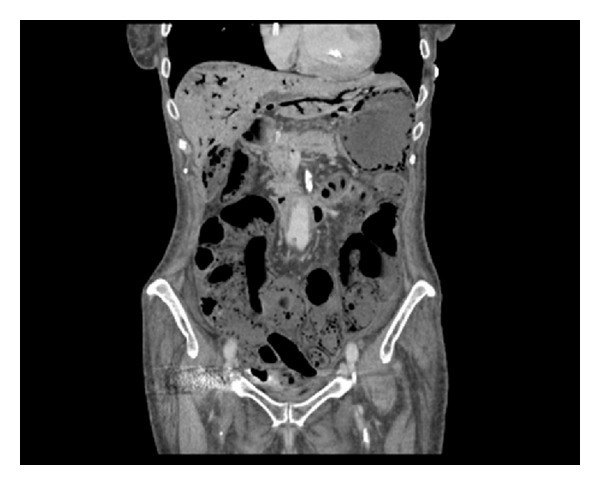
CT showing extensive hepatic portal venous gas, gas within the stomach wall and its draining veins, the gallbladder, common bile duct, and within the majority of the walls of the small bowel (Case 2).
